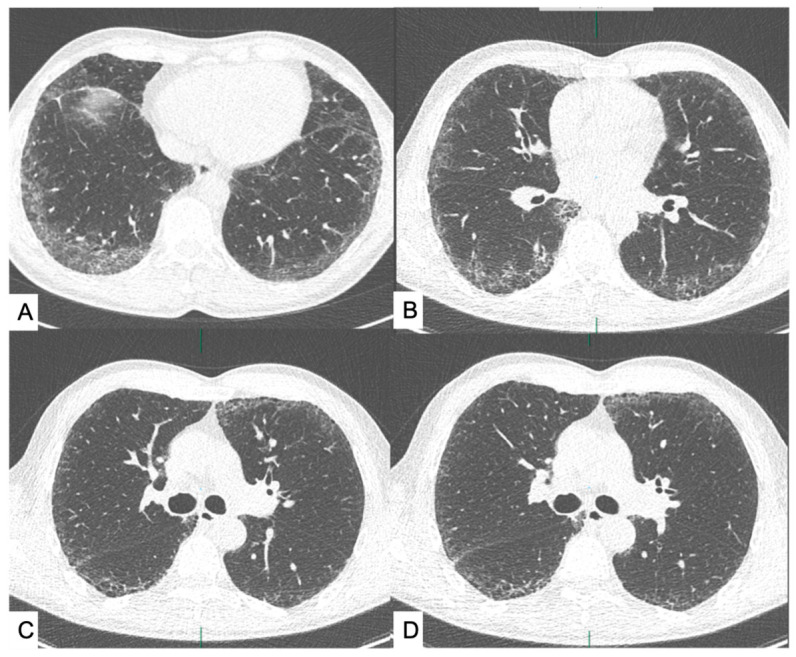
Examples of ILAs defined as non-dependent ground-glass abnormality affecting ≥5% of any lung zone (A) or non-dependent reticular abnormality, honeycombing, traction bronchiectasis, nonemphysematous cysts, and architectural distortion (B–D); low-dose CT axial views.
